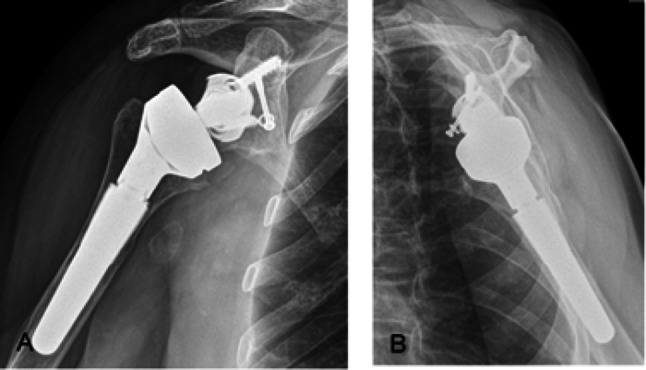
A and B X-ray at 4 years of assessment
